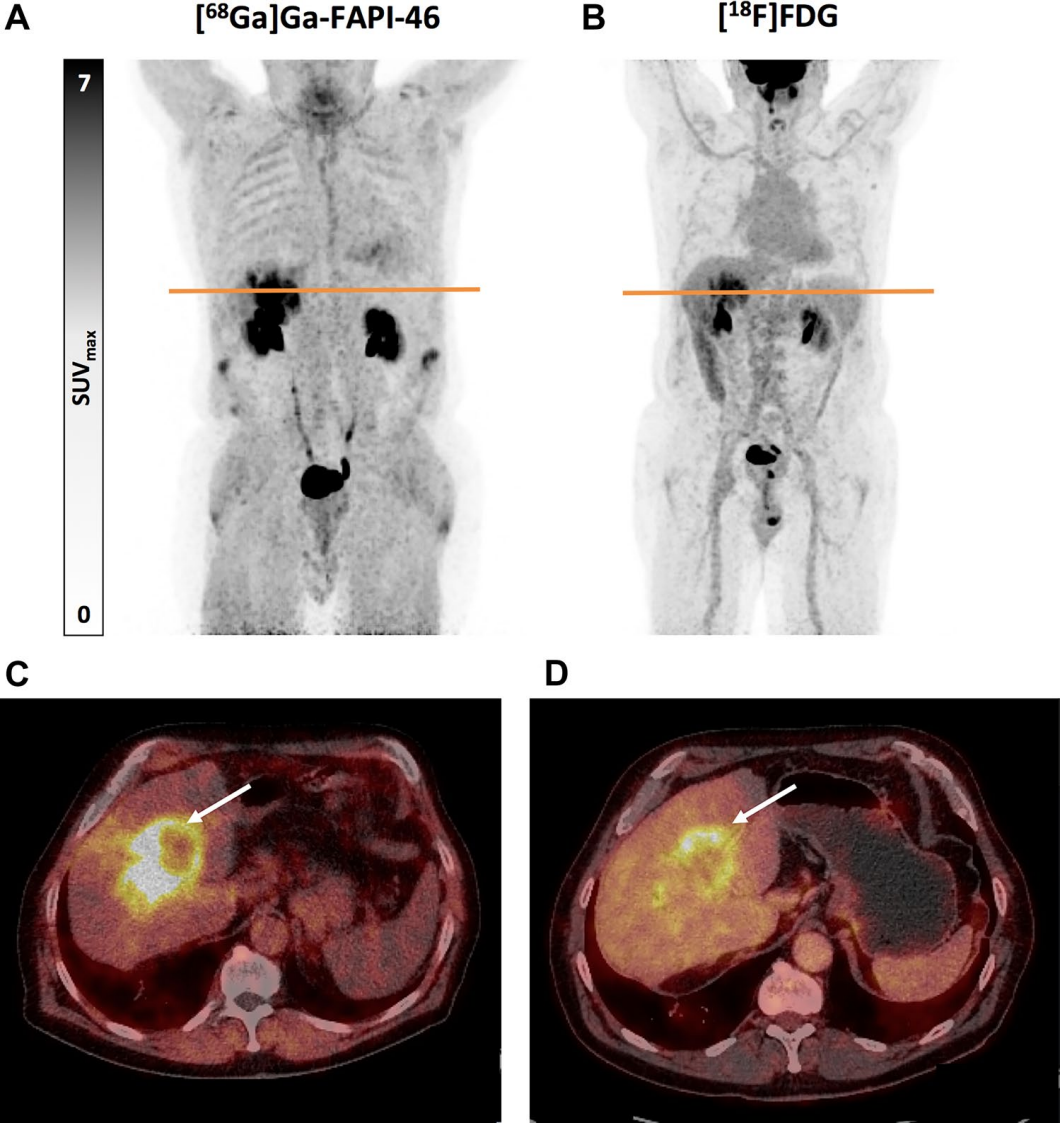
Sixty-seven year old male patient with newly diagnosed intrahepatic cholangiocarcinoma presenting a high tumour uptake on [^68^Ga]Ga-FAPI-46 PET/CT (A and C) and a moderate tumour uptake on [^18^F]FDG PET/CT (B and D), with SUVmax 16.6 and 8.6 for [^68^Ga]Ga-FAPI-46 and [^18^F]FDG, respectively. Maximum intensity projection (MIP) PET images (A and B) and transaxial PET/CT fusion images (C and D) are presented. Transaxial [^68^Ga]Ga-FAPI-46 PET/CT fusion images (C) evidence the high [^68^Ga]Ga-FAPI-46 uptake (white arrow) and the high TBR of the primary tumour with this radiopharmaceutical. In contrast, transaxial [^18^F]FDG PET/CT fusion images (D) show a comparatively higher background uptake and, therefore, a lower TBR, which negatively affects the delineation of the primary tumour
